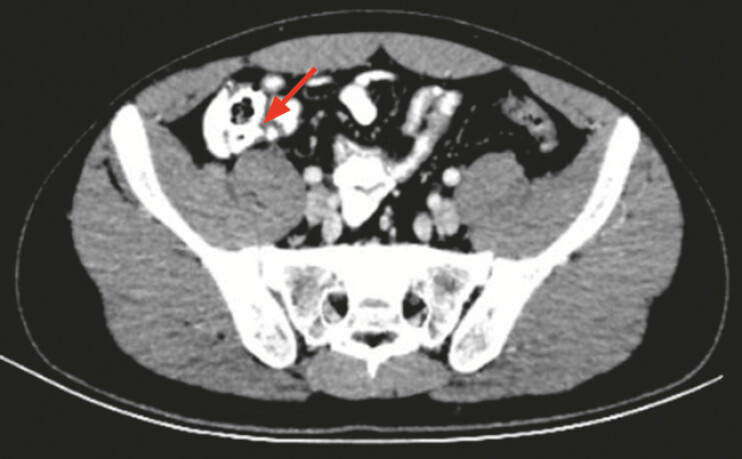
Contrast-enhanced computed tomography showed mild focal thickening of the ileocecal wall without a definite mass (red arrow).
